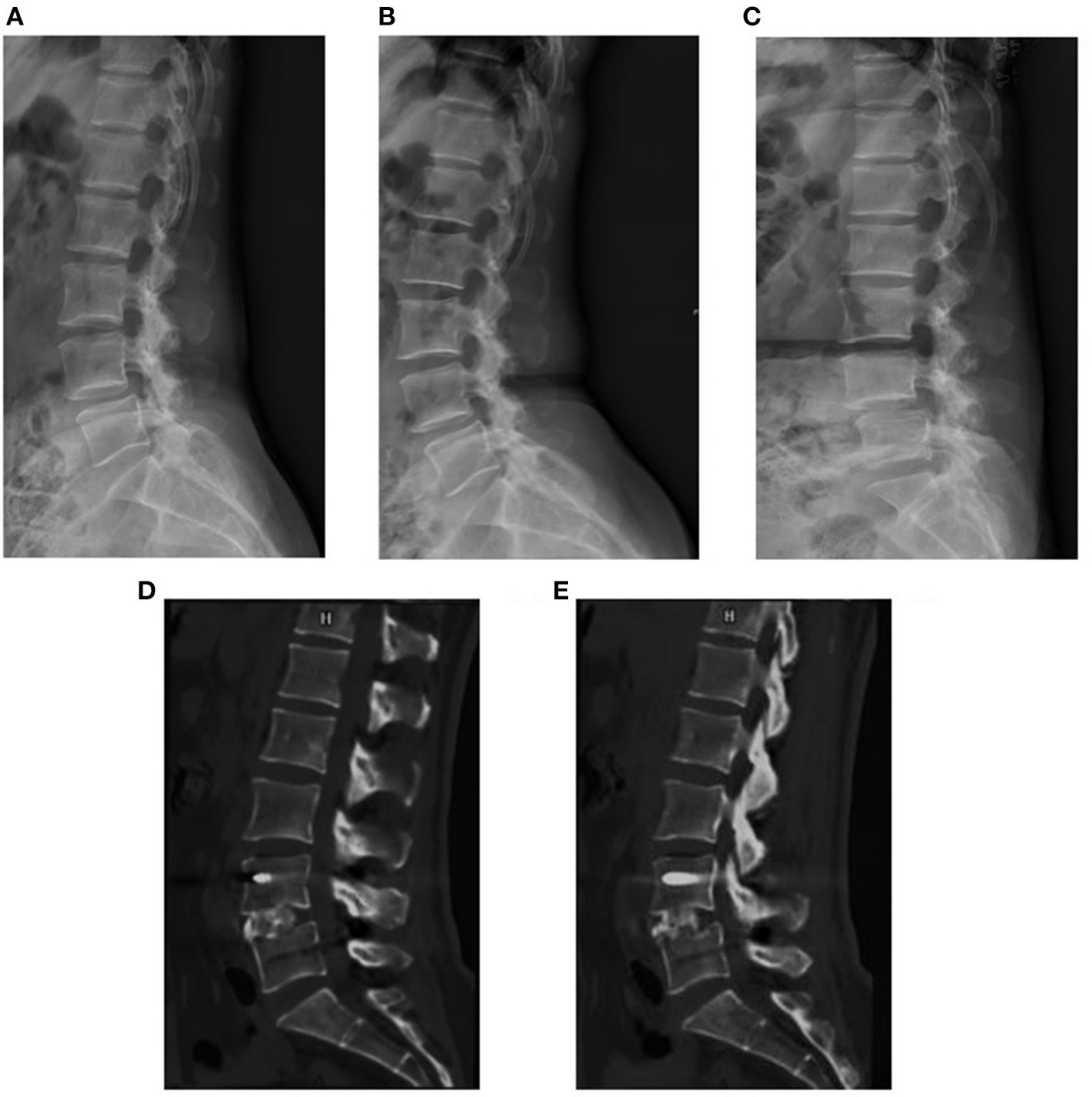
A 48-year-old woman suffered from low back pain for 6 months. The preoperative X-rays showed Meyerding grade I lumbar spondylolistheses at L4 (A–C). The sagittal CT images at 7 months postoperatively suggested solid spine fusion (D,E).
